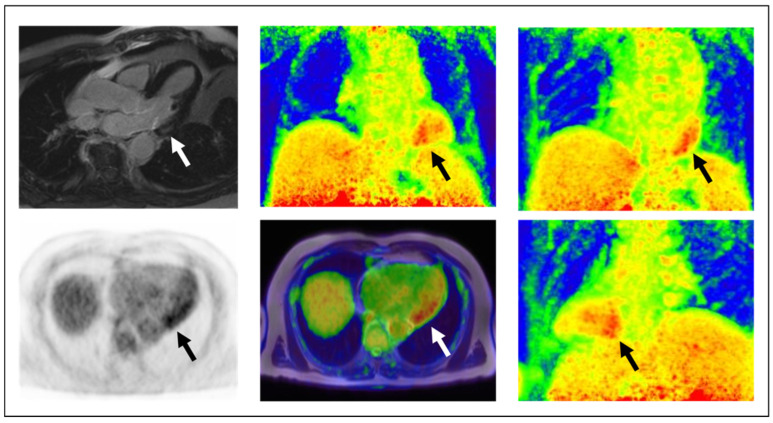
Example of a metastatic malignant melanoma patient under nivolumab treatment in whom checkpoint inhibitor-related myocarditis was verified by ^18^F-FDG PET/MR in our institution. Clockwise from upper-left: Late-phase MR, maximum-intensity-projection PET images from three different angles (anterior, posterior, left-posterior view), axial fusion PET/MR, axial PET. Hybrid imaging showed a hypertrophied normal-sized left ventricle with normal left ventricular ejection fraction and with circumscribed left-ventricular midmyocardial late gadolinium enhancement (infero-latero-basally, white arrow in upper-left image), mild edema, and increased ^18^F-FDG accumulation (black/white arrows in PET image) which was indicative of active myocarditis.
